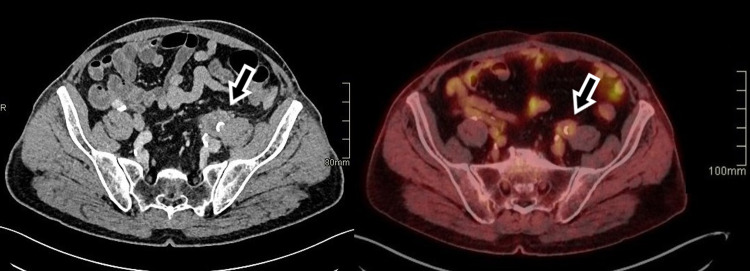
CT of the abdomen in the axial plane when the patient was first admitted to the hospital (A), and a follow-up PET-CT in the axial plane after two months of treatment (B). The retroperitoneal fibrosis is marked with arrows, showing a decrease in mass size. CT: computed tomography; PET-CT: positron emission tomography-computed tomography
